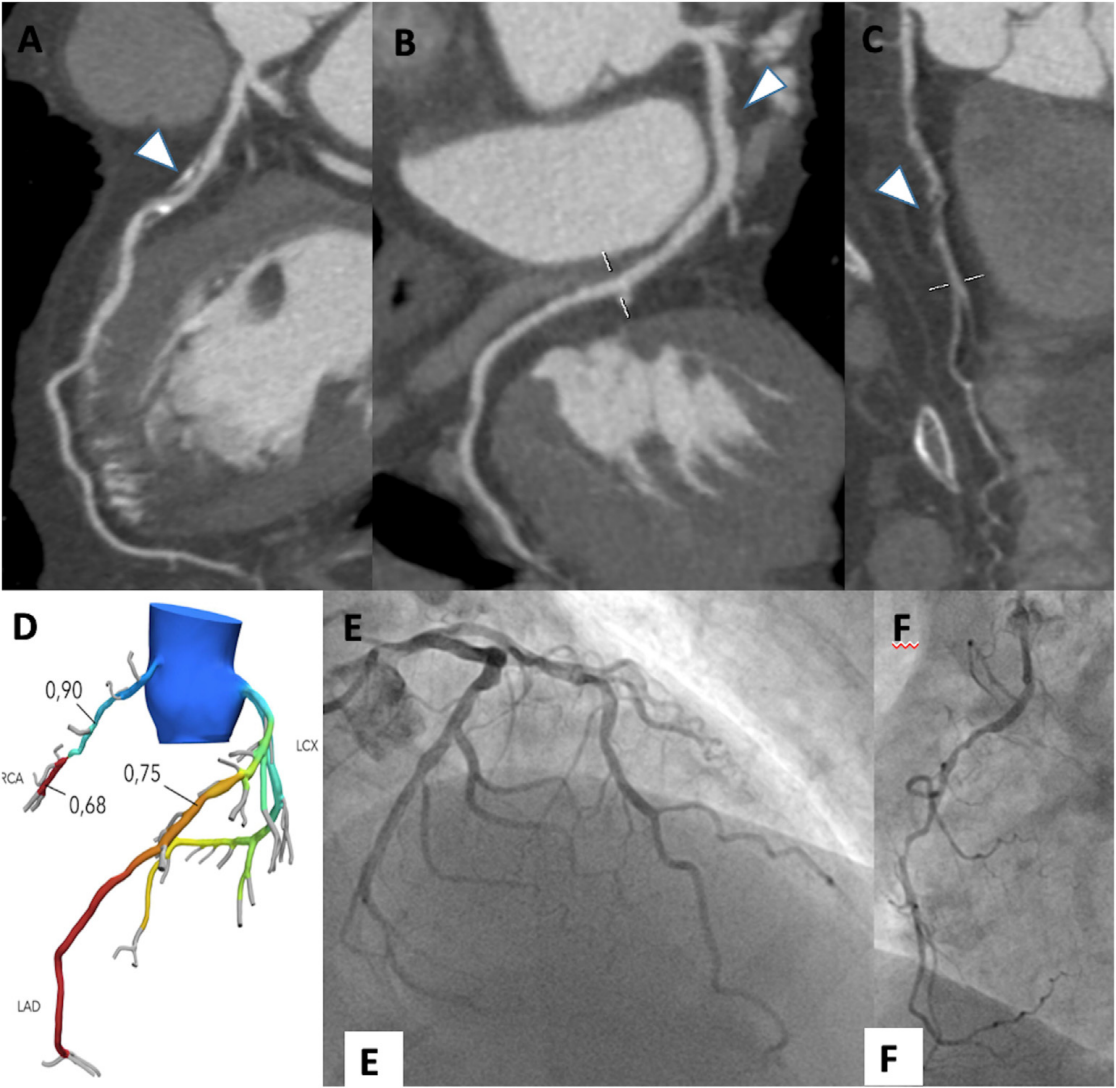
70-year-old male patient underwent to coronary computed tomography for chest pain. Left anterior descending artery shows a severe mixed plaque stenosis (A, arrowhead); left circumflex artery shows a moderate proximal fibro-lipid plaque (B, arrowhead) while right coronary artery shows a severe fibro-fatty plaque stenosis (C, arrowhead). The FFR_ct_ assessment confirmed the functional significance of the stenosis on left anterior descending artery and right coronary artery (D), while FFR_ct_ values of the left circumflex artery were above the ischemia threshold of 0.80. The invasive coronary angiogram shows severe stenosis of the mid segment of left anterior descending artery (E) and mid segment of right coronary artery.
